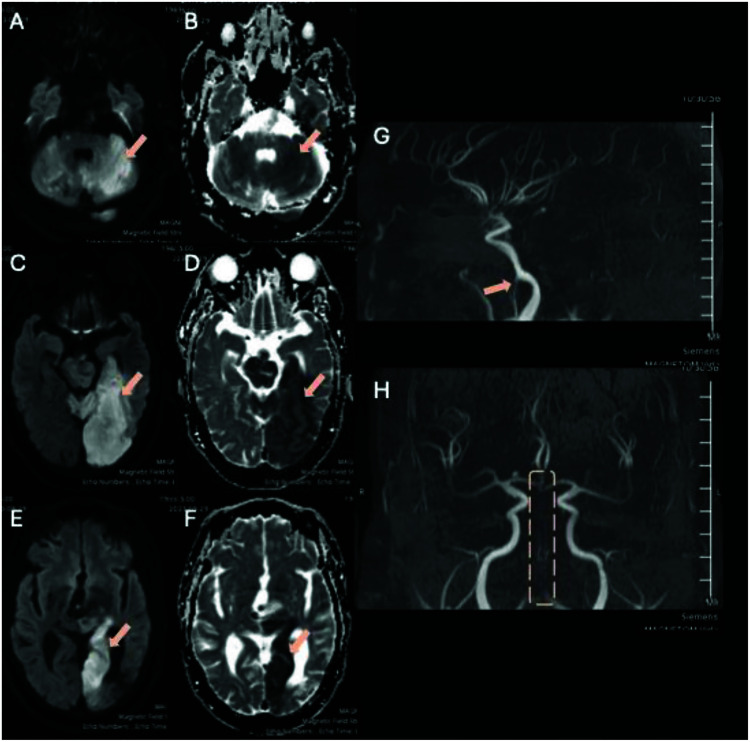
Magnetic resonance imaging (MRI) of the brain with magnetic resonance angiography (MRA). (A, C, E) Brain MRI showing a large area of diffusion restriction in the left occipital lobe extending into the temporal lobe and left thalamus, along with bilateral cerebellar infarcts, more prominent on the left, with involvement of the vermis. (B, D, F) Corresponding findings are seen on the apparent diffusion coefficient (ADC) maps. (G, H) Cranial MRA showing non-visualization of the distal vertebral arteries, basilar artery, and posterior cerebral arteries (PCAs), with preserved visualization of the distal internal carotid arteries (ICAs), anterior cerebral arteries (ACAs), and middle cerebral arteries (MCAs).
